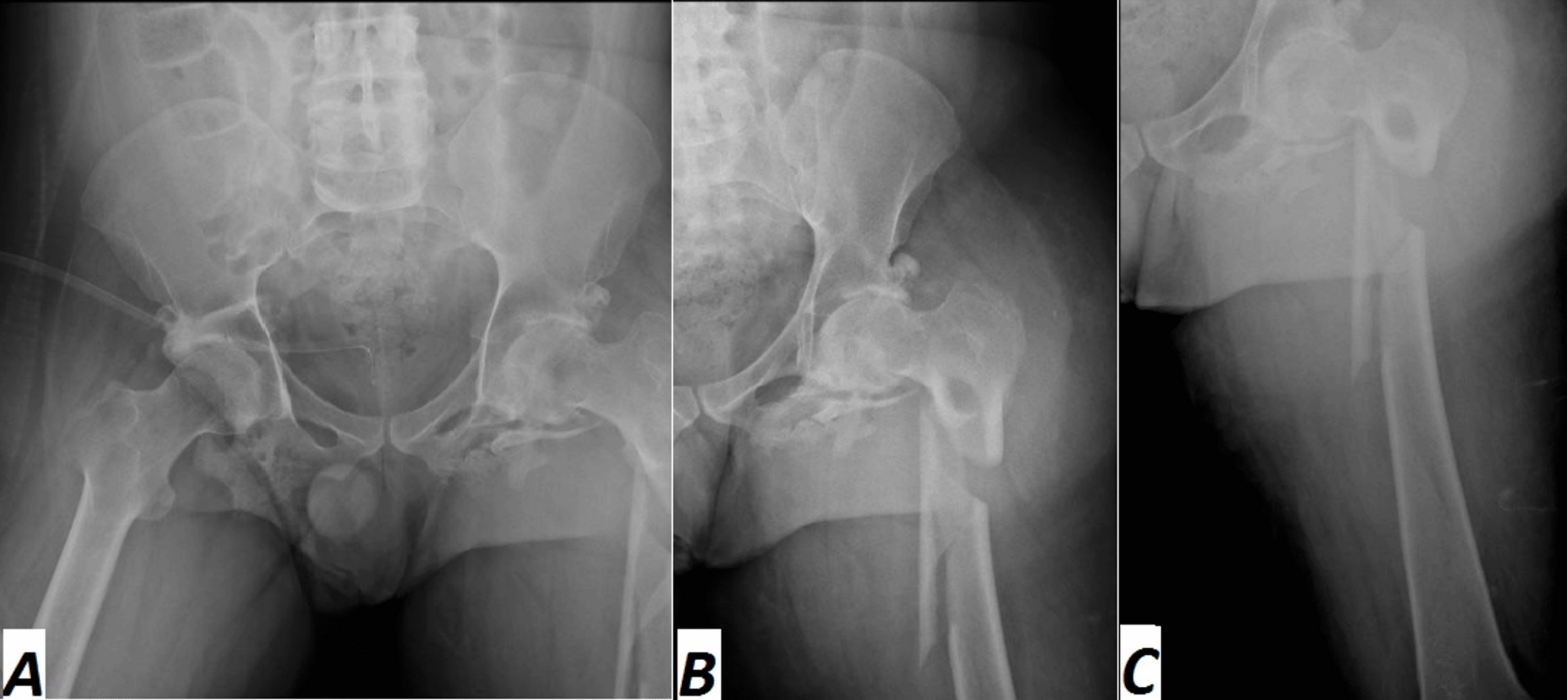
Preoperative (A) anteroposterior and (B) lateral radiographs show a closed, severely displaced, comminuted subtrochanteric left femur fracture.
